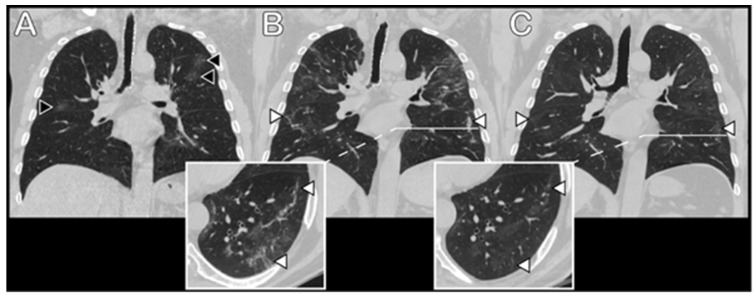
Coronal reconstructions and axial thin-section chest CT images of a 70-year-old man with confirmed COVID-19 pneumonia. (A) First CT scan 4 days after the onset of symptoms in the early stage of disease. Mild randomly distributed ground-glass opacities (black arrowheads) can be seen in both lungs. (B) CT scan at a later stage of disease (27 days after the onset of symptoms). Progression of previously observed ground-glass areas, as well as new areas of opacification can be seen. A reticular pattern emerged, with some parenchymal bands in peripheral parts of the lungs. (C) Follow-up CT scan 2 months later. A decrease in the density of ground-glass opacities can be seen, with only a slight decrease in the area of lesions. Some parenchymal bands persisted, with a decrease in abundance and thickness (white arrowheads).
